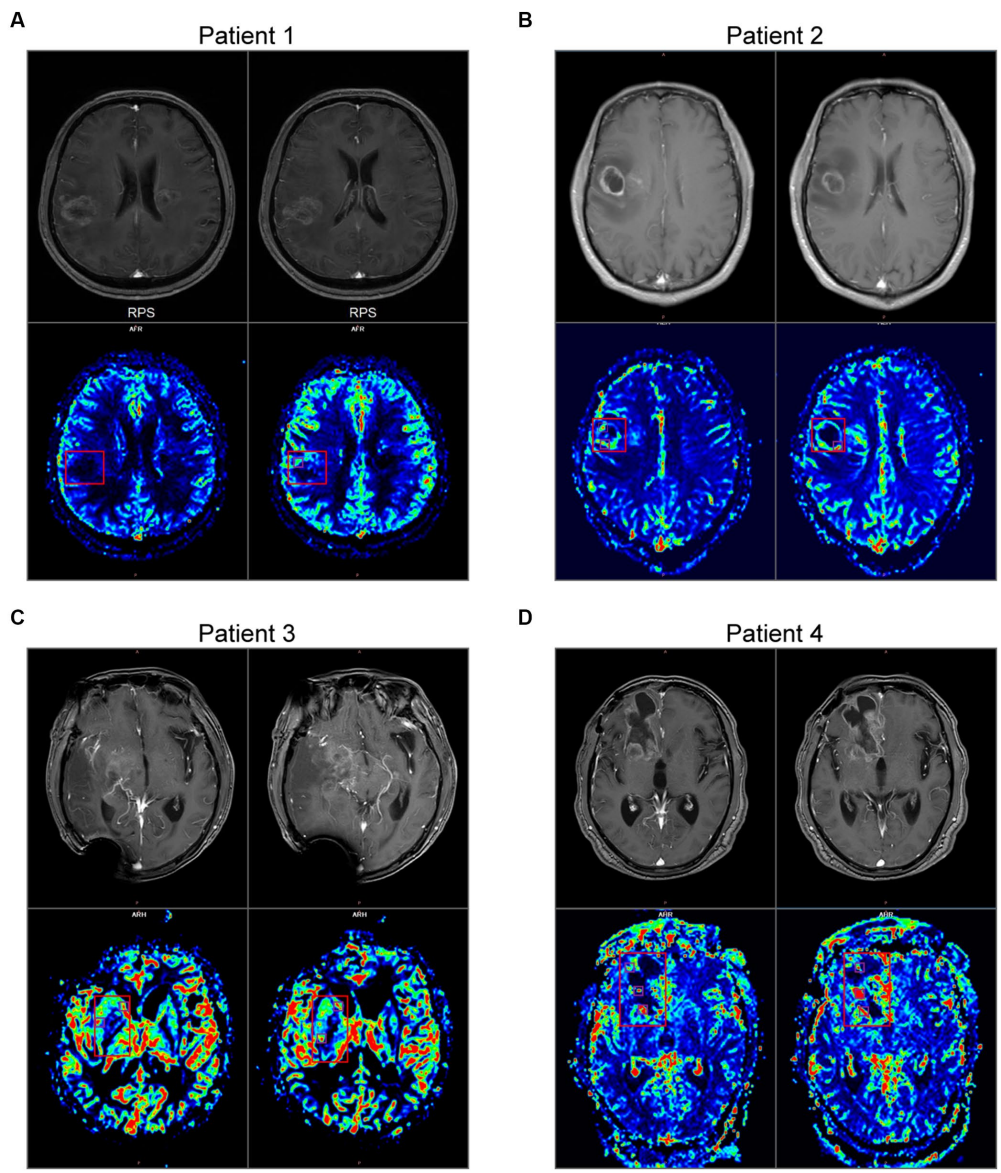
MRI and PWI images of 4 gliomas. (A,B) Imaging characteristics of primary glioma. Upper: the images of T1W + C. Bottom: the images of CBF in PWI. (C,D) Imaging characteristics of recurrent glioma. The main body of the glioma is marked with a red box, and the areas of relatively high CBF values at the peritumoral brain zone are marked with purple boxes.
